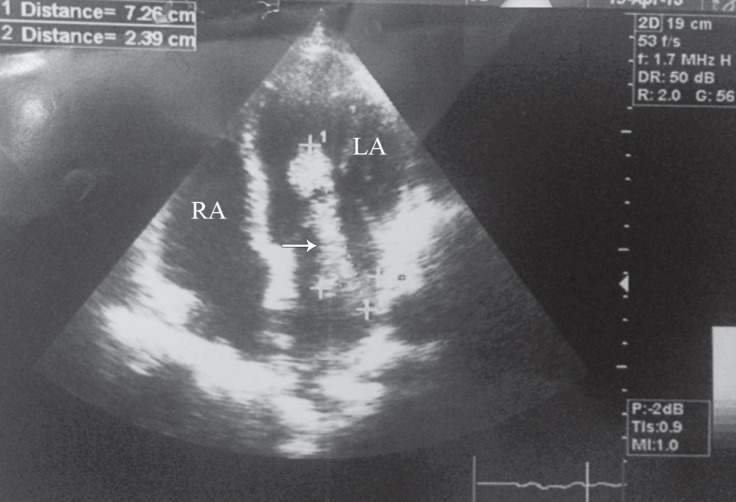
Transthoracic echocardiogram reveals the presence of a large mobile mass in the left atrium protruding into the left ventricle (arrow).
